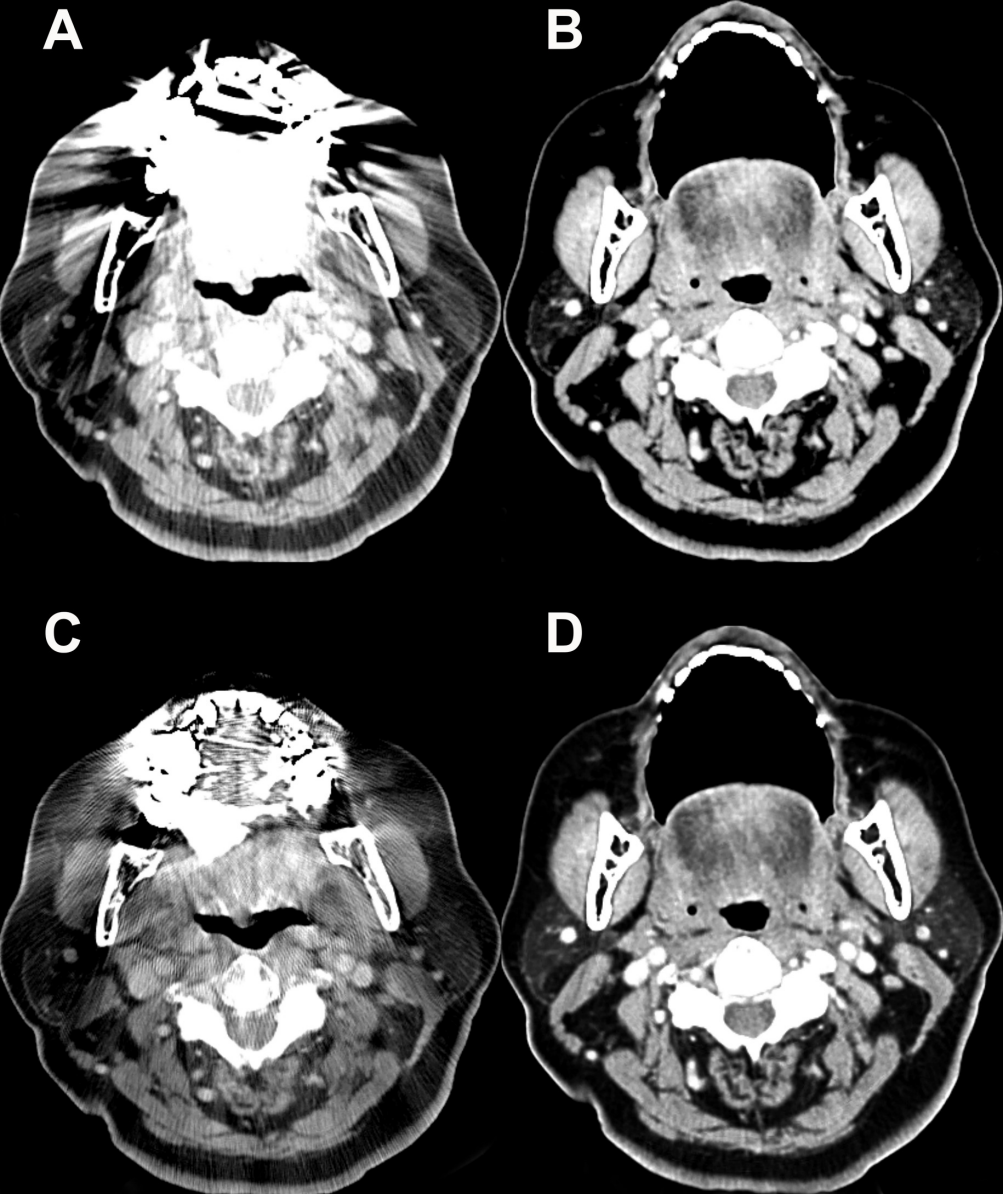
Post-contrast Computed Tomography (CT) images of a 71-year-old woman with a dental prosthesis. Bilateral palatine tonsils are completely covered by the metallic artifact from the adjacent dental prosthesis on a conventional CT image (A). However, on the open mouth CT image (B), there is no streak artifact obscuring the bilateral palatine tonsils, because the axis of the streak artifact from the dental prosthesis is tilted. On a conventional CT image with O-MAR processing (C), the bilateral palatine tonsils are partly seen with decreased artifacts, but the image texture becomes blurry. The added effect of O-MAR is not noticeable on the open mouth CT image with O-MAR processing (D) since the streak artifact is already displaced from the open mouth maneuver. O-MAR = metal artifact reduction for orthopedic implants.
